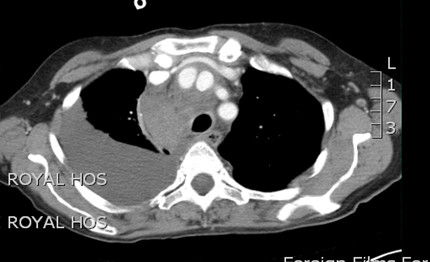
CT scan shows contrast-filled innominate artery false aneurysm next to innominate artery proper, right hemothorax and extensive superior mediastinal hematoma causing tracheal deviation to the left.
